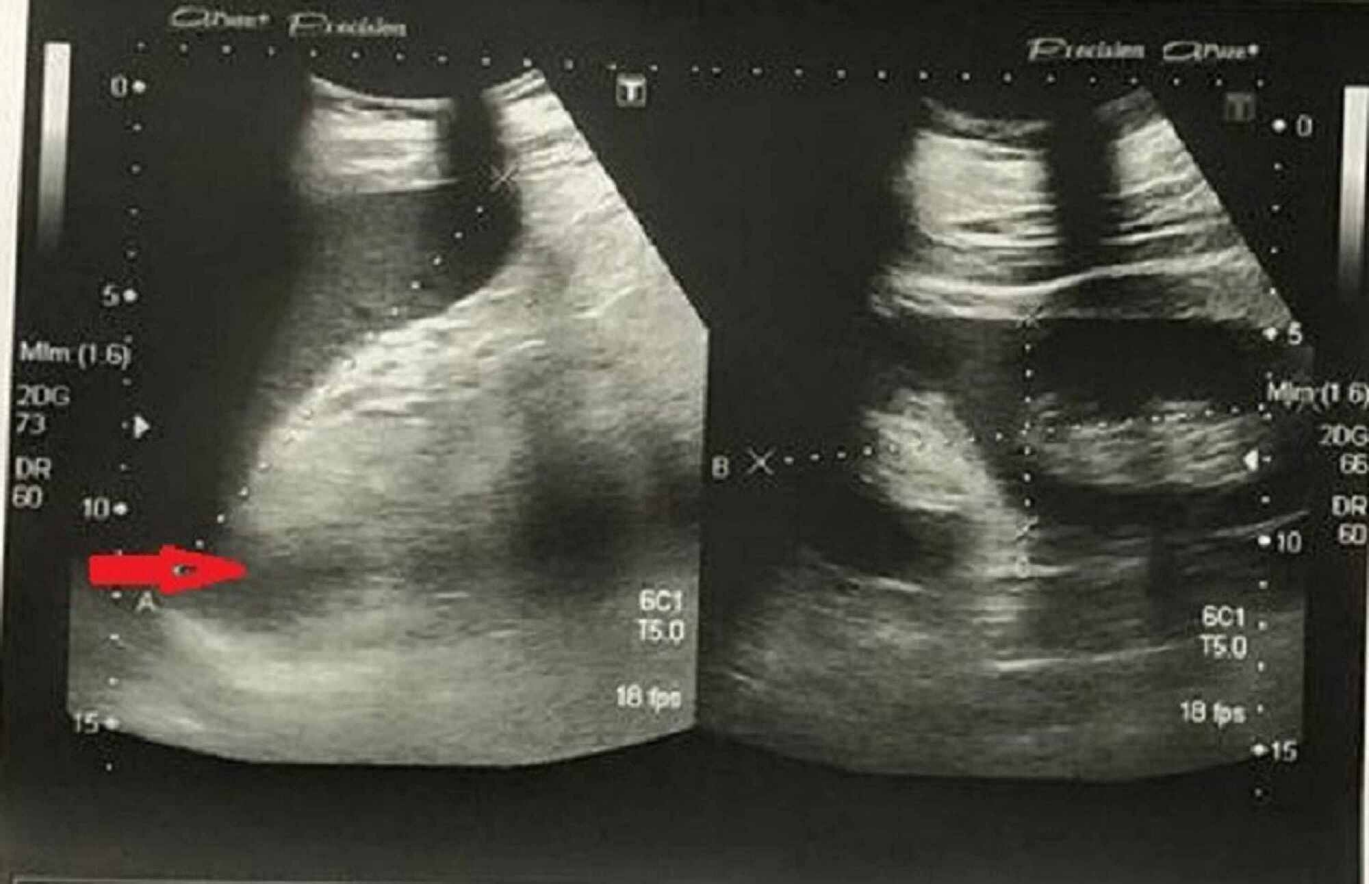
Ultrasound abdomen shows fluid levels (red arrow)
